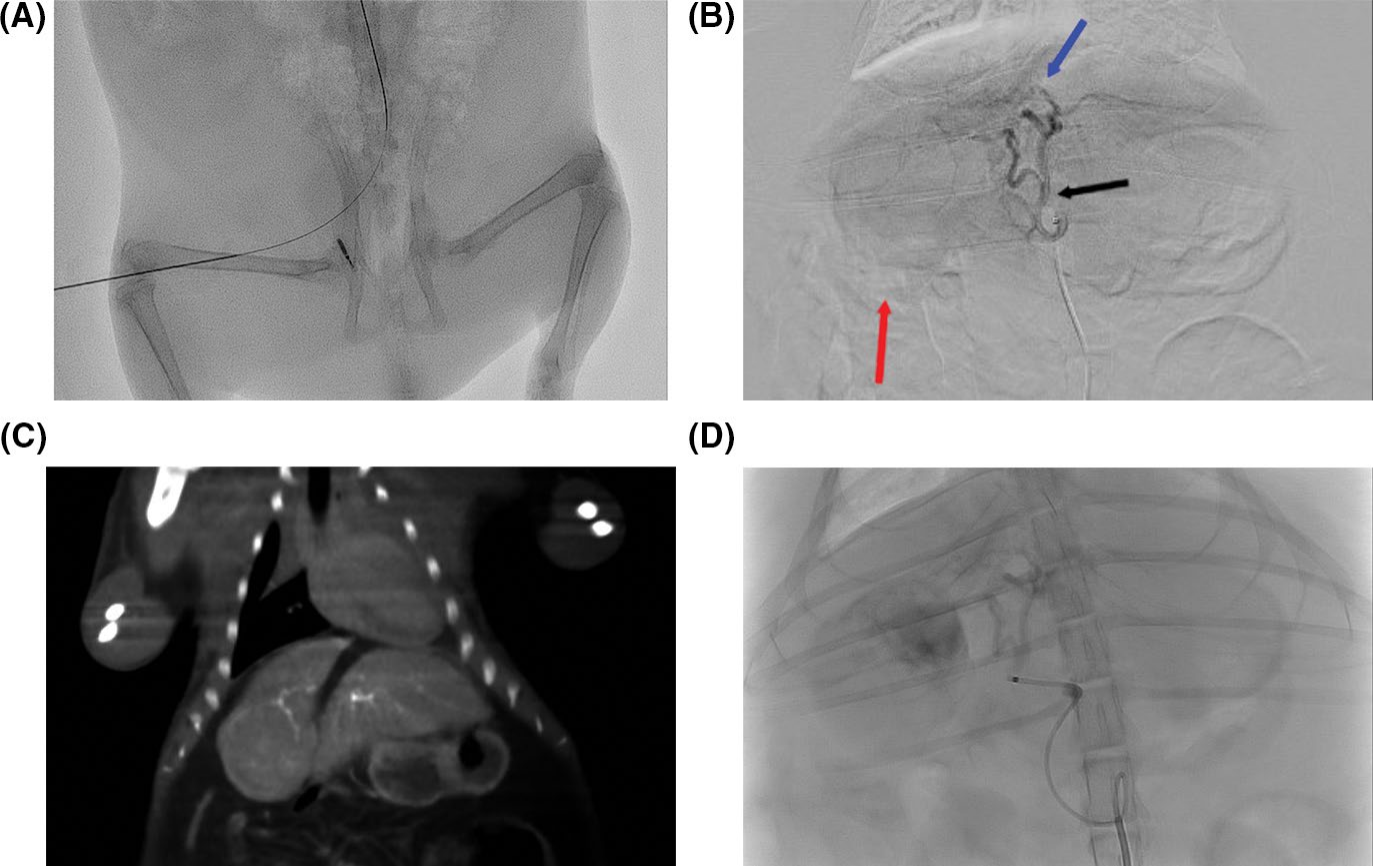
Angiographic findings. A, Spot image after microwire advancement subsequent to obtaining percutaneous arterial access. B, Angiogram with the catheter tip in the common hepatic artery. Black arrow denotes the bifurcation of the right and left hepatic arteries. Red arrow points to outline of the right lobe HCC with peripheral regions of enhancement. Blue arrow points to a hypertrophic and tortuous segment 4 artery which provided the dominant arterial supply to tumor. C, Coronal‐reconstructed CBCT image demonstrating tumor enhancement. The tortuous, hypertrophic arterial feeder is demonstrated in the superior aspect of the tumor. D, Post‐embolization of the tumor from the hepatic proper artery demonstrates contrast retention in the superior aspect of the tumor
